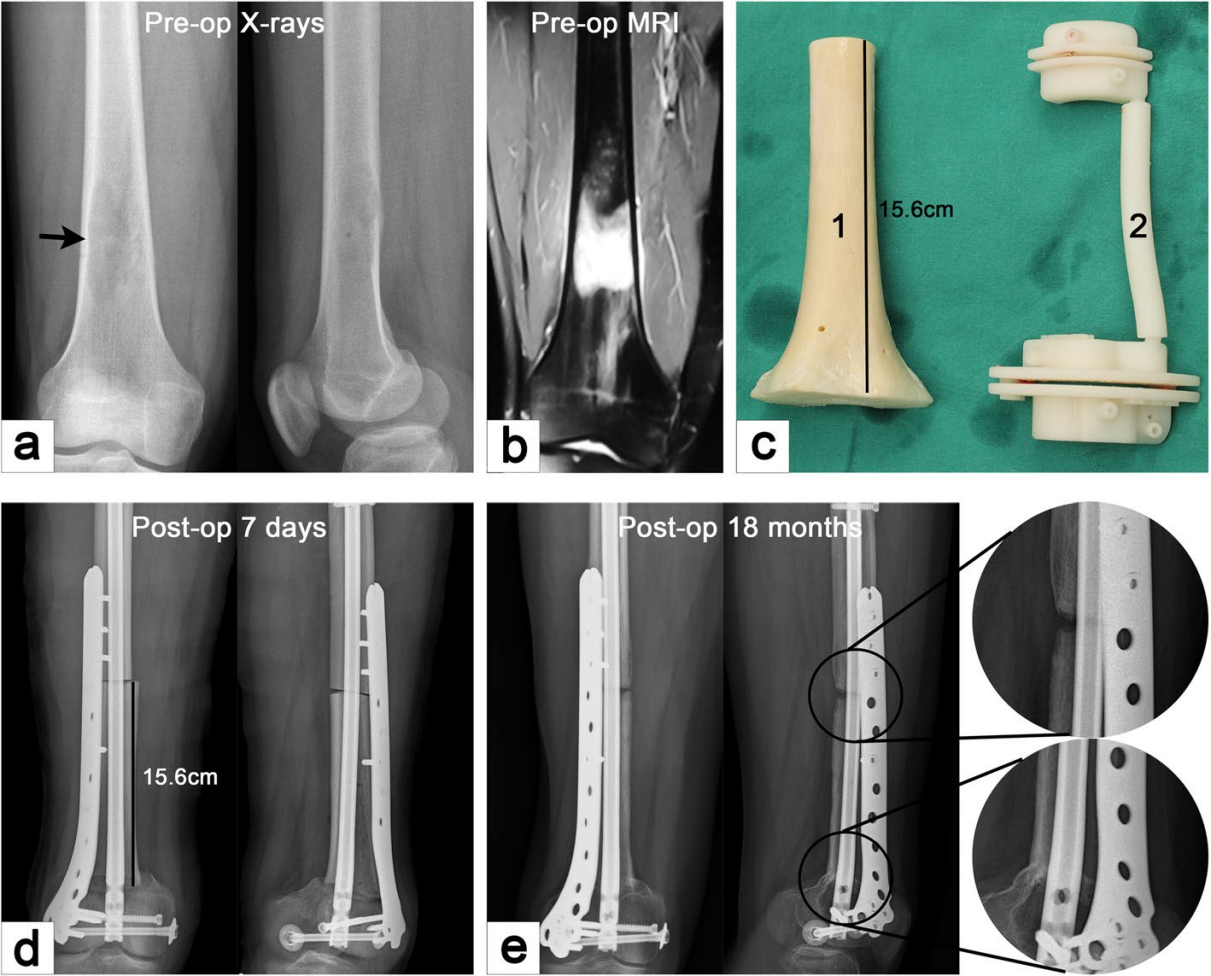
Case 21. A case of stage IIA osteosarcoma in distal femur. a and b Anteroposterior and lateral X-ray and MRI images. c Allograft for repairing bone defect and 3D-printed osteotomy guide plate. d and e Anteroposterial and lateral X-ray were performed at 7 days and 18 months postoperatively, respectively. The results showed that the host–donor junction of the metaphysis had achieved bone healing, but the junction at the diaphysis had nonunion, and there was no sign of loosening of internal fixation
